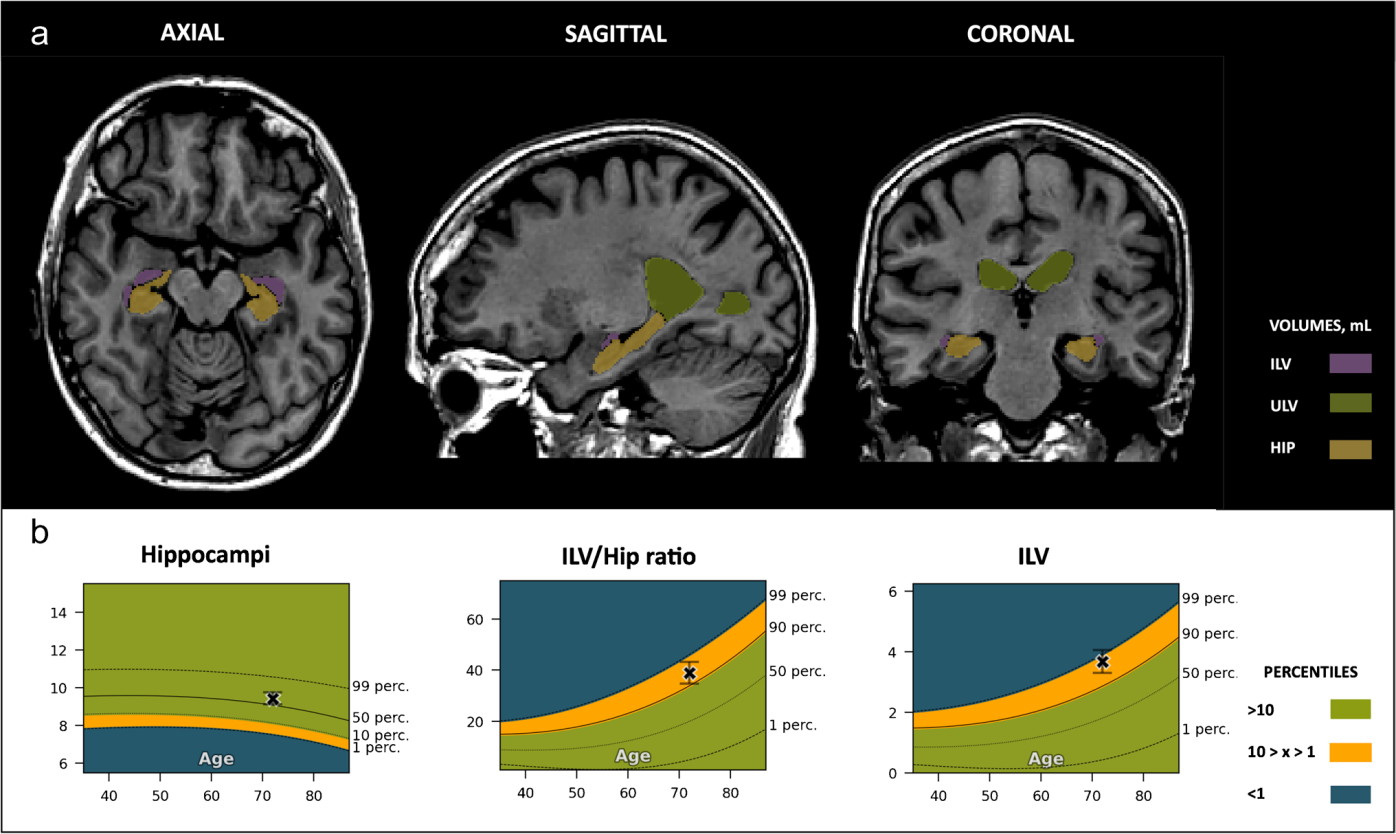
Mild cognitive impairment (MCI) case. Cross-sectional coronal T1-weighted image at the level of the medial temporal lobe and hippocampus with segmentation of the upper lateral ventricles (green), inferior lateral ventricle (purple), and hippocampus (yellow). A — Automated volumetric measurements *left*: axial view; *middle*: sagittal view; *right*: coronal view. B — Population graphs *left*: hippocampal volumes of this subject showing the gray “x” marker in the blue region, below the 1st percentile; *middle*: the ILV/Hip ratio of this subject showing the gray “x” marker in the blue region, just above the 99th percentile. *Right:* ILV volumes of this subject showing the gray “x” marker in the orange region, above the 90th percentile. Color-coded population graph percentile interpretation; 1–90th percentile, green (normal); 90–99th percentiles, orange (caution); > 99 th percentile; dark blue (abnormality)
